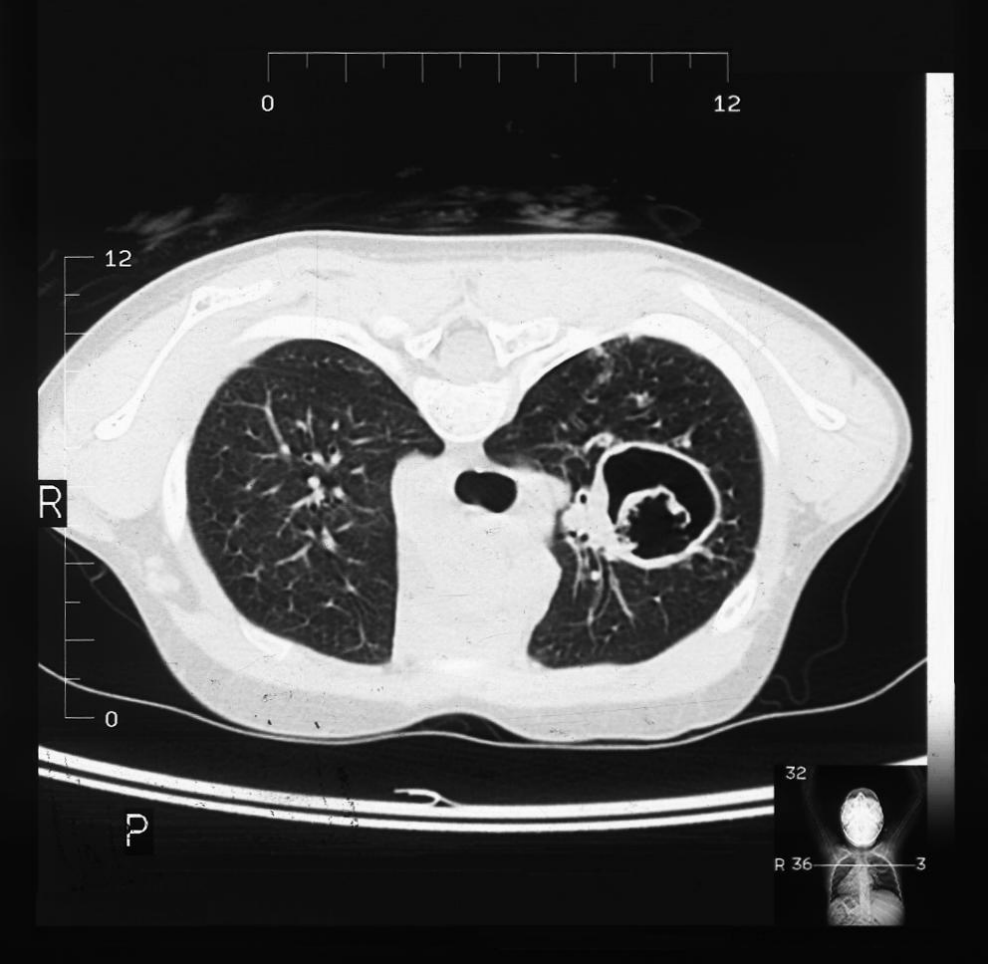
High-resolution computed tomography of the chest in a child with HIES, showing aspergilloma in a postinflammatory cavity.
